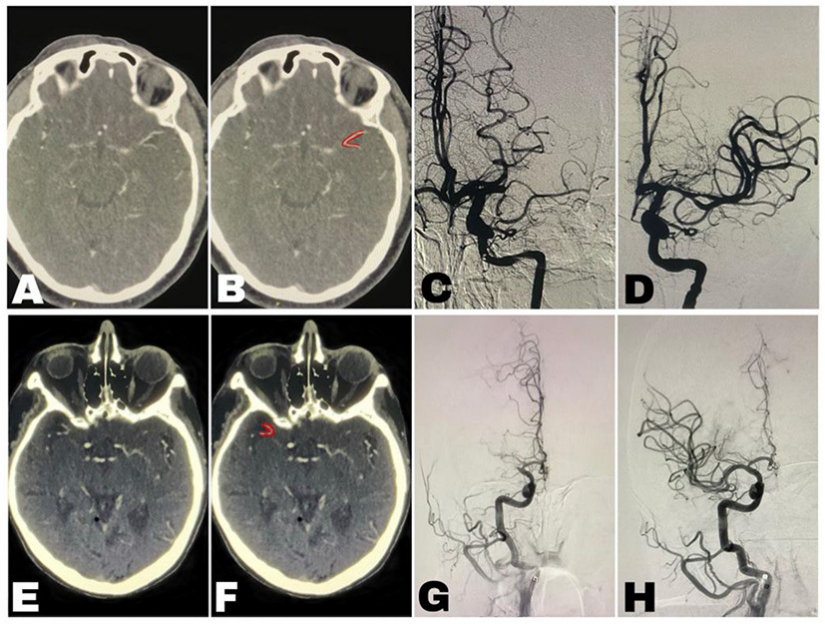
Imaging of two patients [(A–D) patient-1, (E–H) patient-2]. (A,E) The “Tilted-V Sign” unmarked. (B,F) The “Tilted-V Sign” (marked in red)—axial images of CT angiogram of the head showing filling of both proximal M2 segments of the Middle Cerebral Artery (MCA) distal to an occluded, not visualized M1-MCA segment, thereby creating a Tilted-V shape. (C,G) Digital subtraction angiography (DSA) before endovascular thrombectomy (EVT) showing complete occlusion of the M1-MCA segment but without visualization of vessels distal to the occlusion. (D,H) DSA following EVT showing successful recanalization.
